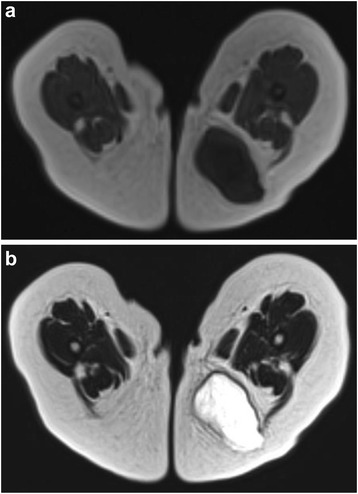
MRI images of the left buttock mass. a T1-weighted axial image showing a subcutaneous encapsulated mass measuring 4.6 × 2.9 × 3.9 cm. The mass was mostly homogenous with low-intensity signals. b T2-weighted axial images showing that the mass yielded mostly high-intensity signals
